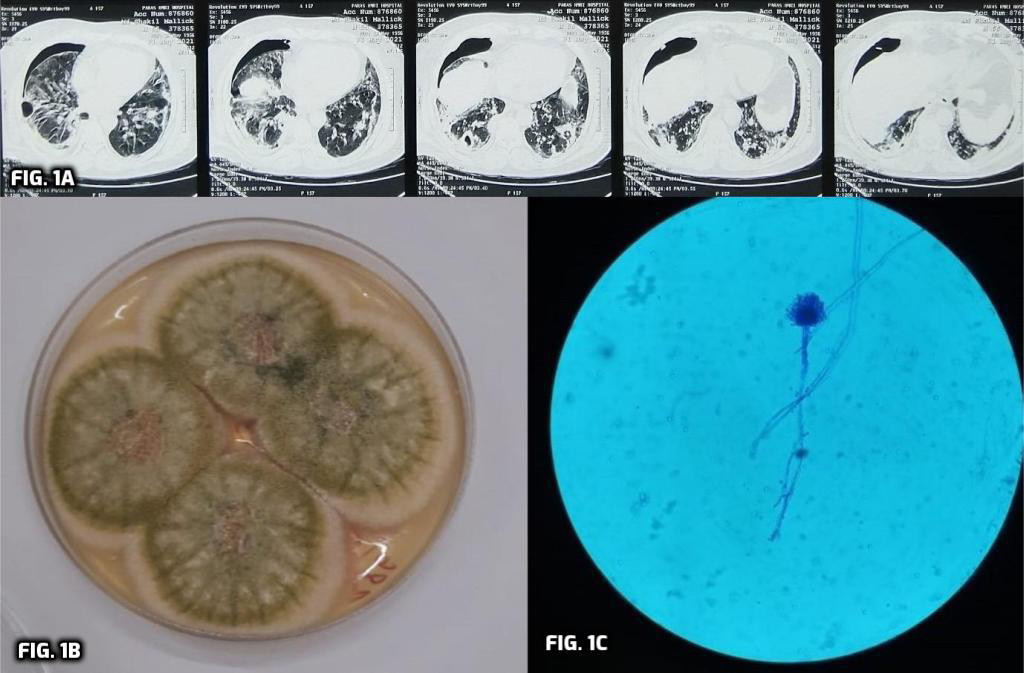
(a) Multiple thick-walled peripheral cavities in bilateral lung parenchyma, diffuse patchy consolidation in bilateral lower lung lobes and hydropneumothorax in the right pleural space (chest HRCT image). (b) *Aspergillus flavus*. The obverse side of the culture plate shows a velvety, yellow to green colony. (c) The LPCB mount showing biseriate phialides covering the entire vesicle.
